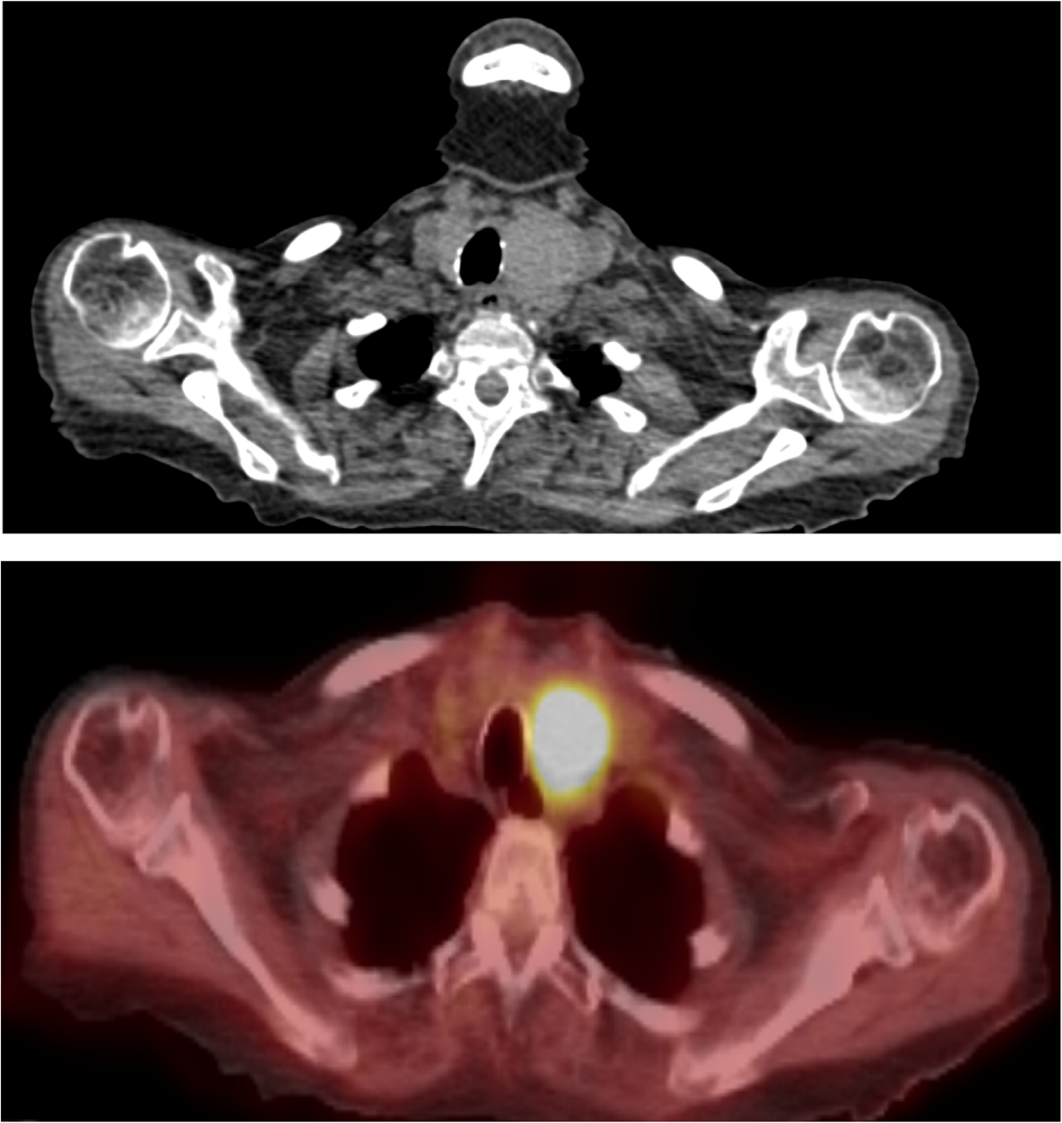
Seventy-nine-year-old female with a past medical history of systemic sclerosis and Raynaud’s disease. She presented with symptoms of weight loss, retrosternal chest discomfort and general cognitive decline. Prior investigations had shown a raised CRP and normal WCC in her routine blood, and an OGD showed oesophagitis only. She had a CT CAP and PET-CT to investigate for possibility of an organ tumour/large vessel vasculitis. Image a shows axial CT with a large left-sided thyroid mass. Image b is a fused axial PET-CT scan showing 33 mm × 36 mm metabolically active lesion (SUV max 10.3 g/ml) which occupies much of the left lobe of the thyroid gland and is responsible for tracheal deviation to the right. FNAC confirmed follicular carcinoma
